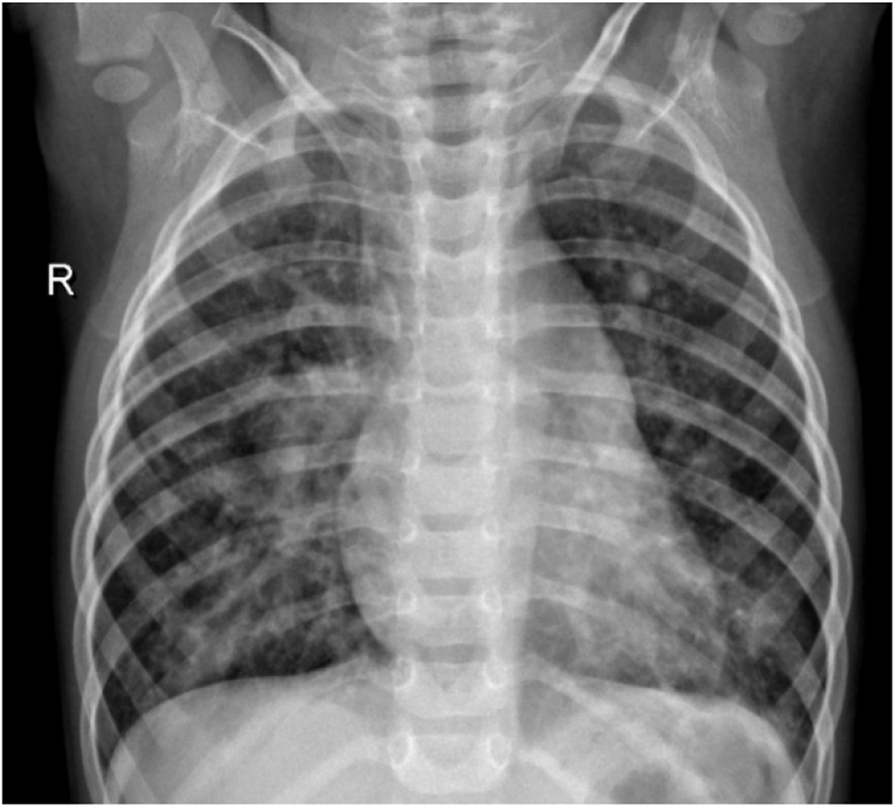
Sample of chest X-ray image of a non-Covid VP patient
